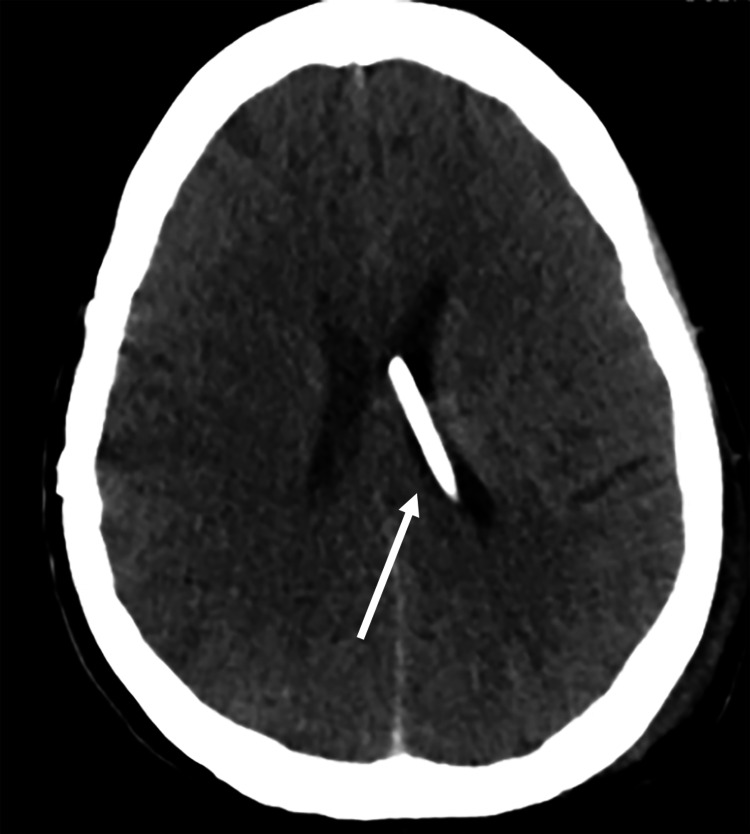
Axial CT without contrast showing good position of the VP shunt catheter with no evidence of acute hemorrhage or ischemia. VP: Ventriculoperitoneal
